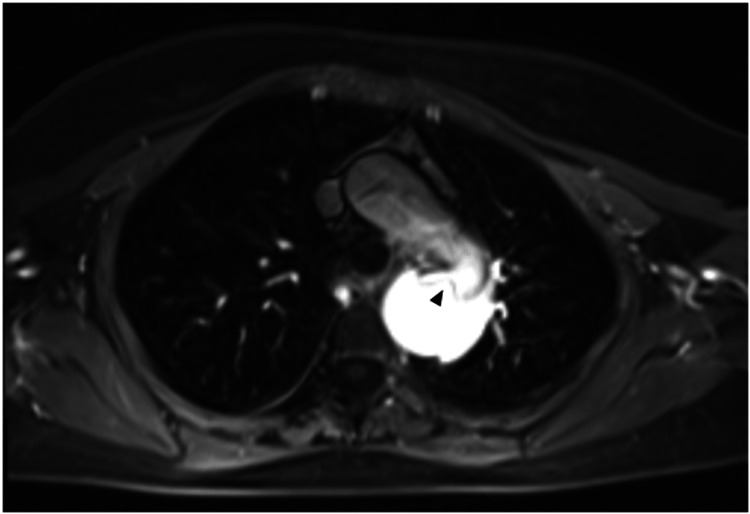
MRI with gadolinium enhancement showing a flap (arrowhead) at the beginning of the descending aorta.
